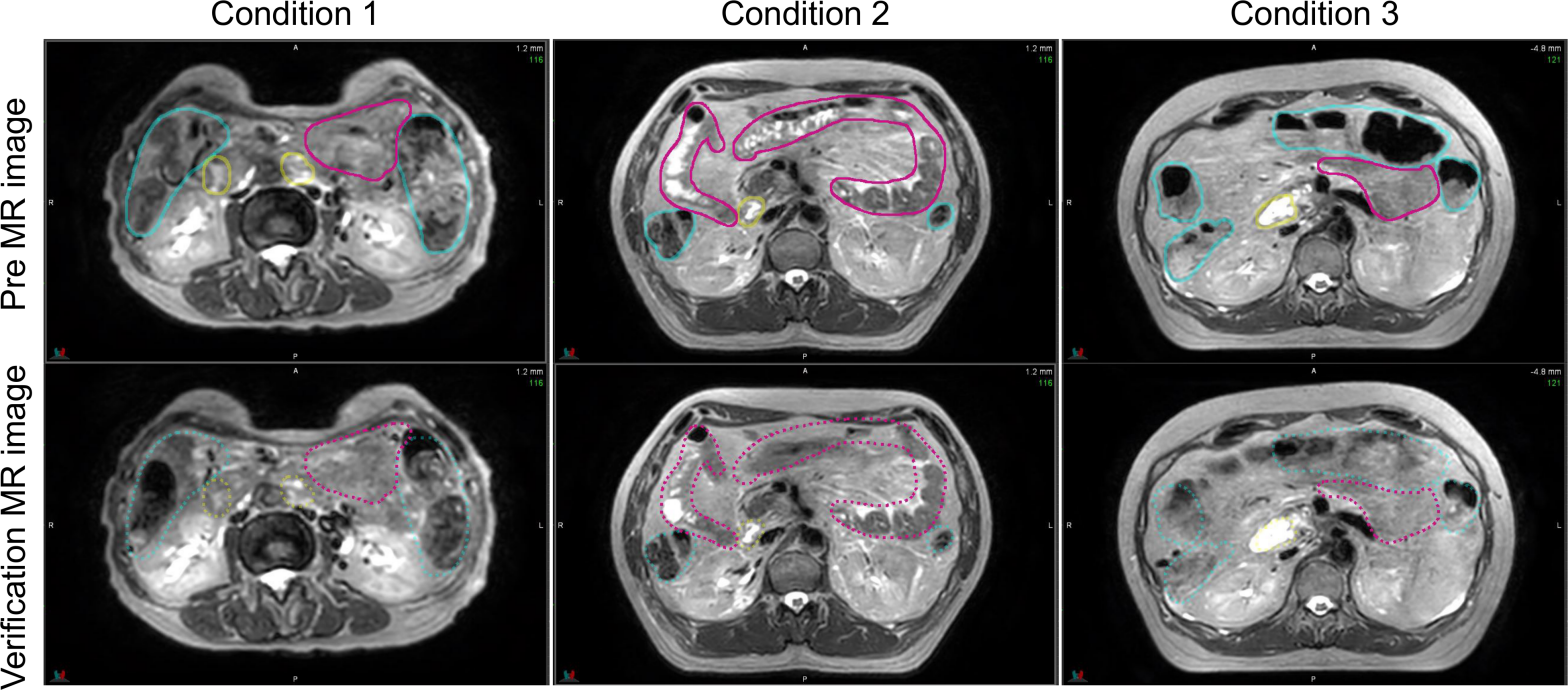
Comparison of the pre- and verification MR images for conditions 1, 2, and 3. The contour created in the pre-image is depicted on both MR images. MR, magnetic resonance.
